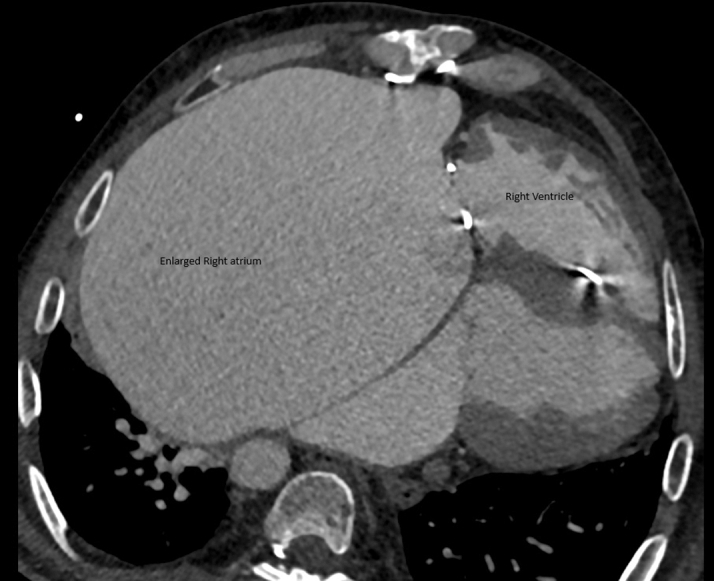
Cardiac Computed Tomography Axial Cut Demonstrating Markedly Enlarged Right Atrium
